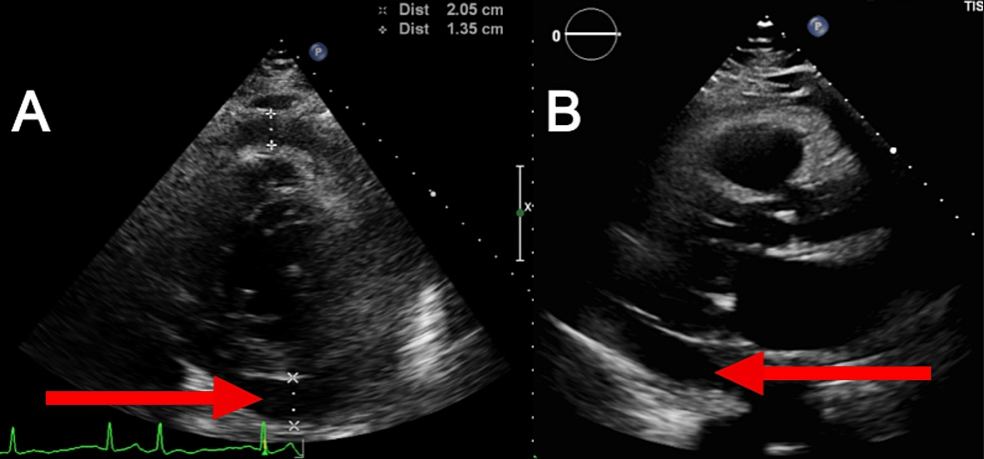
Echocardiogram of the patient A. Echocardiogram demonstrating pericardial effusion (red arrow) in the parasternal short-axis view. B. Echocardiogram demonstrating large pericardial effusion (red arrow) in the parasternal long-axis view
